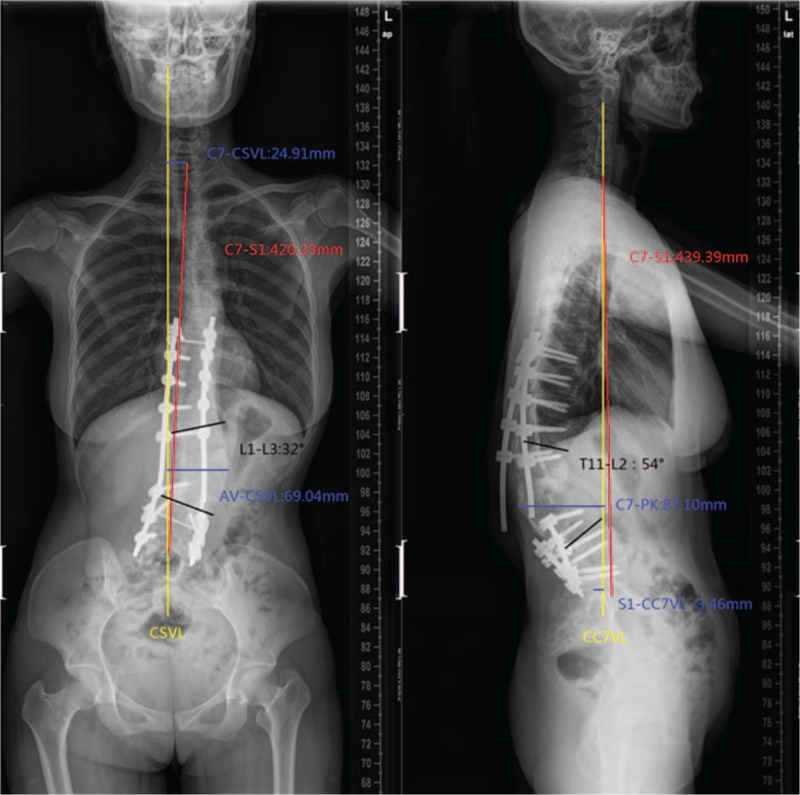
Lateral and anteroposterior X-ray, the rods were broken, kyphoscoliosis had increased.
